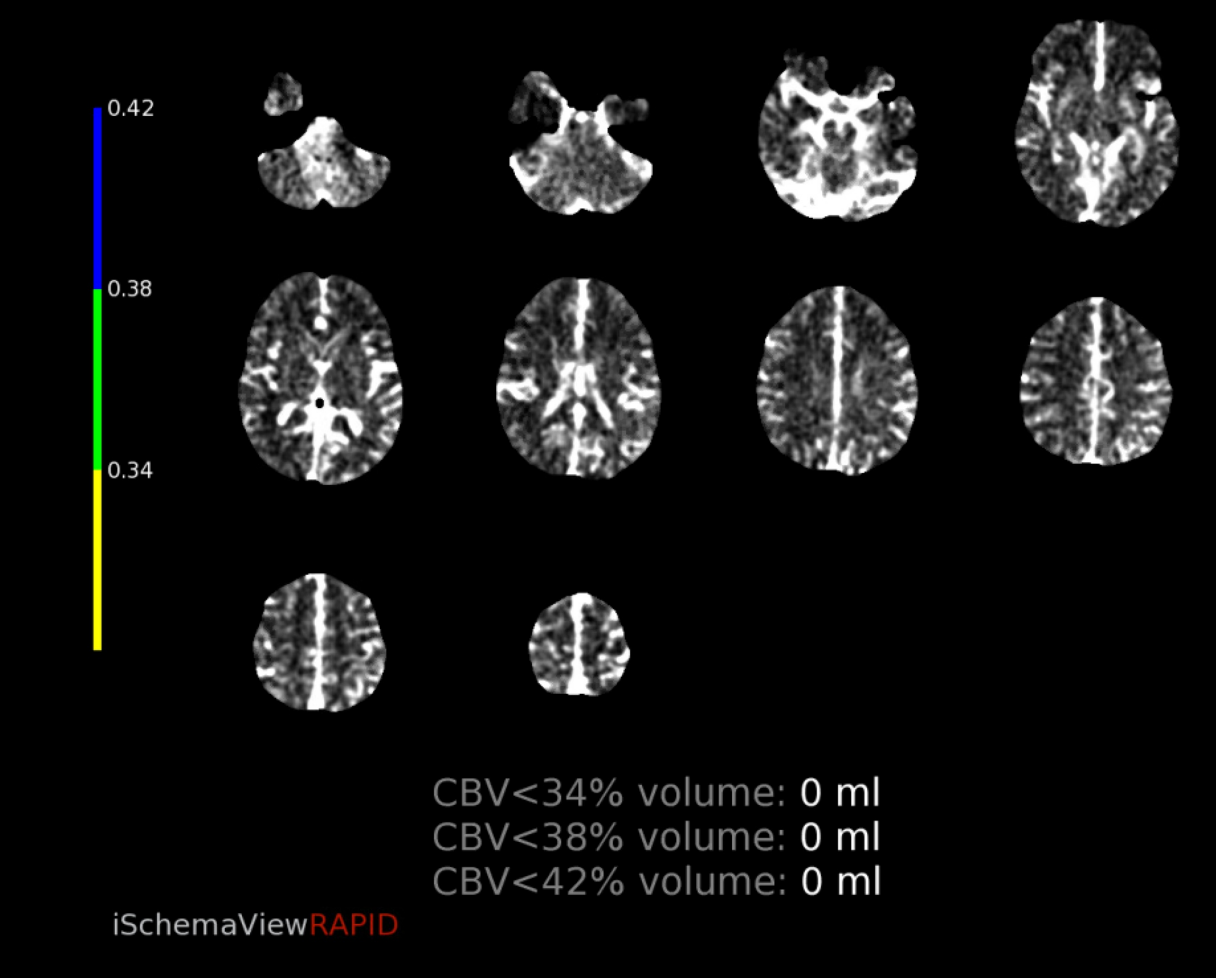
CT perfusion demonstrating normal CBV CBV=Cerebral blood volume
